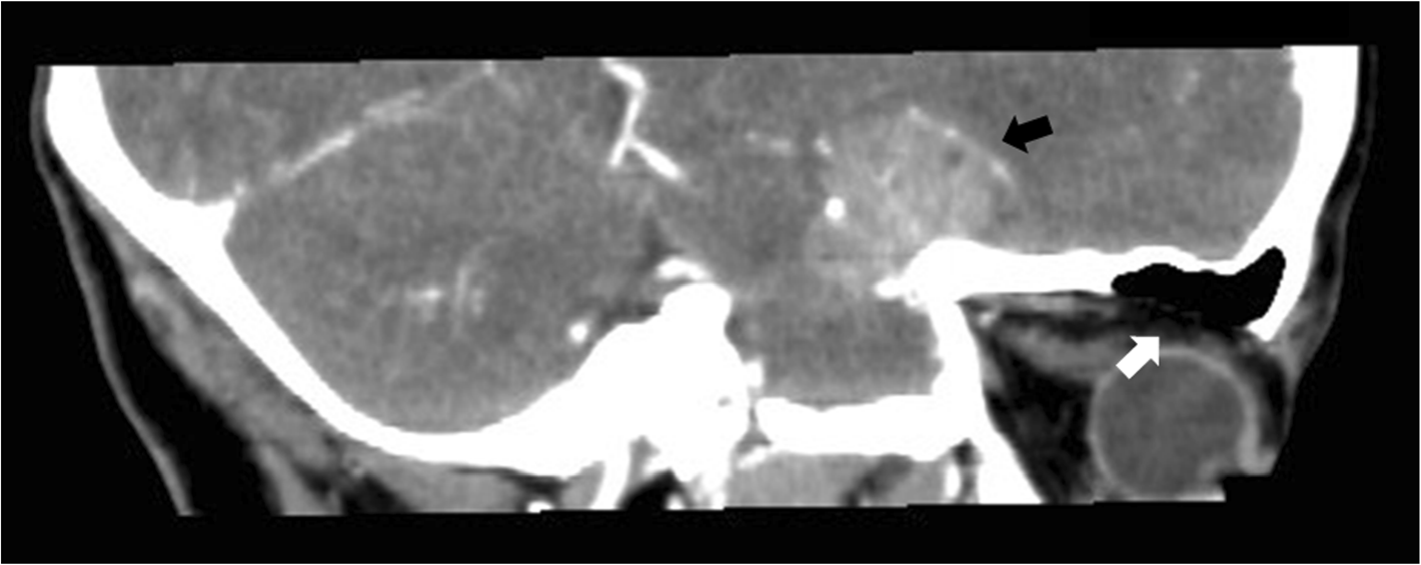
Preoperative brain CT: a bone defect has been found in the basal frontal sinus (white arrow), which might be the pathway the bone wax migrated to the orbit. Black arrow: the mass in sellar-suparsellar-parasellar region before the craniotomy
